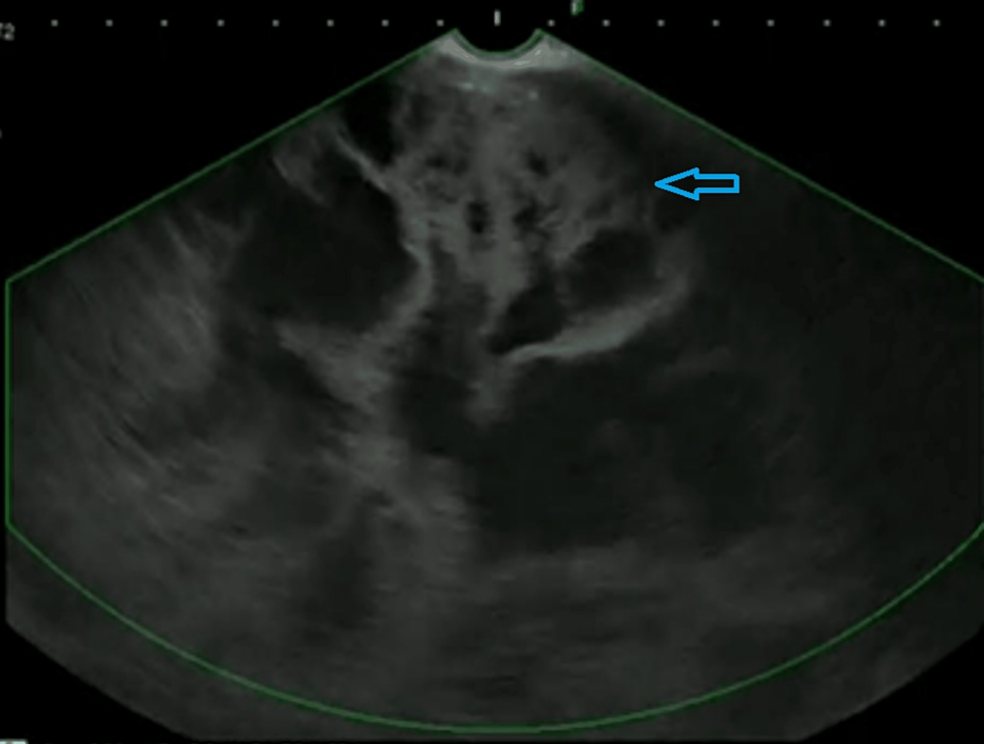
Endoscopic ultrasound image Endoscopic ultrasound showed a rounded retrogastric mass with thickened wall. The content was both cystic and solid with mobile vegetation.
